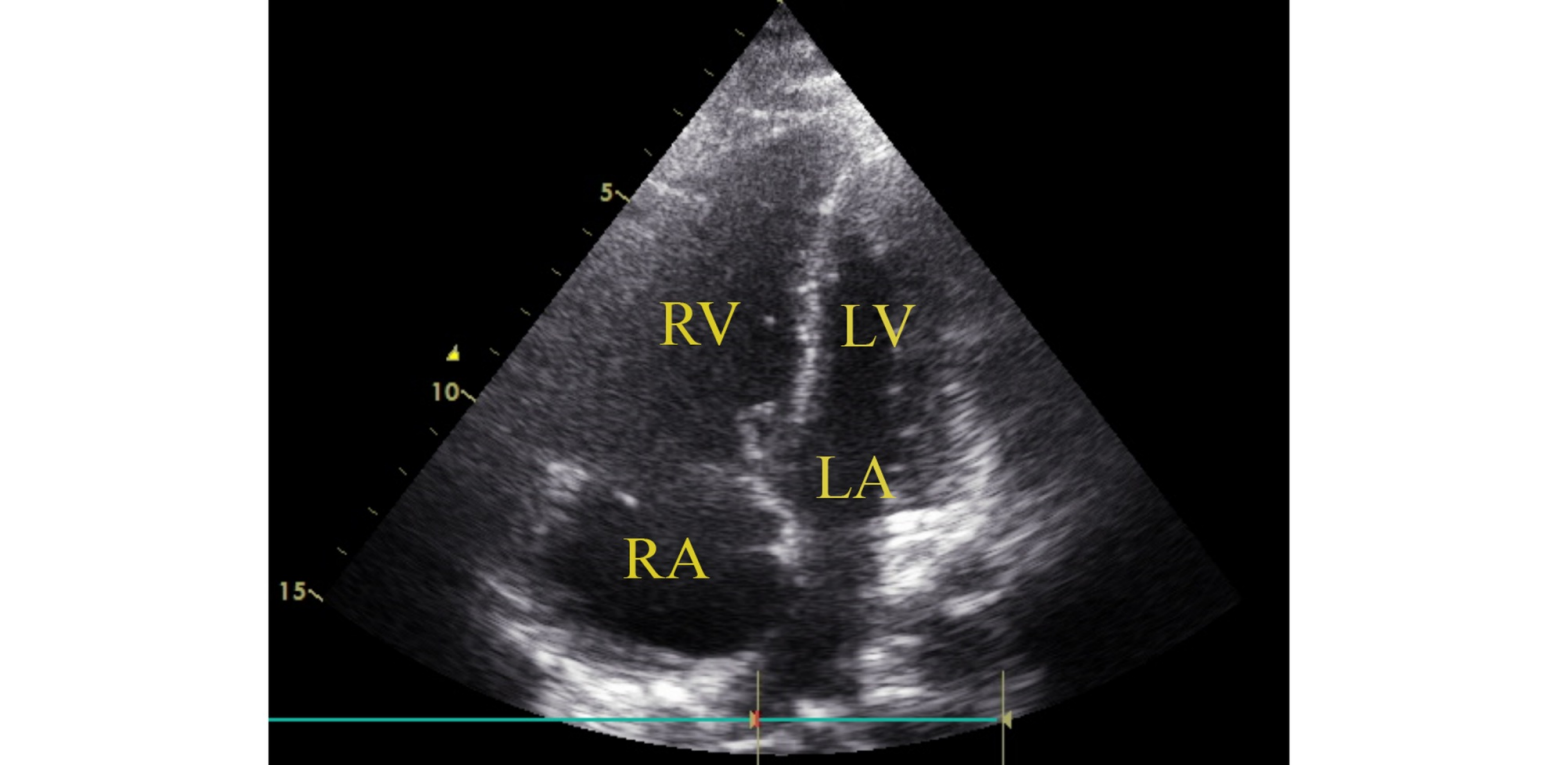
Echocardiography shows acute cor pulmonale. Hugely dilated right side of the heart. Dilated right ventricular dimension, with reverted Brenheimic effect and paradoxical septal motion. Normal left ventricular chamber internal dimensions with good systolic function. No regional resting wall motion abnormalities. RV, right ventricle; LV, left ventricle; RA, right atrium; LA, left atrium
